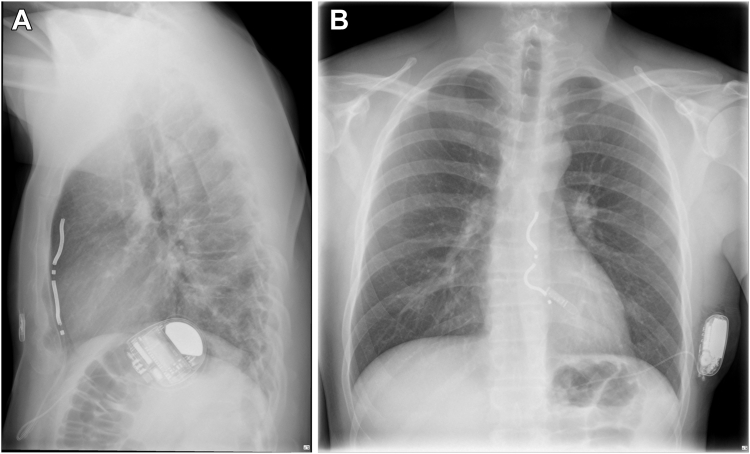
Radiograph after implant position lead and device of an Aurora extravascular implantable cardioverter-defibrillator in anteroposterior and lateral positions.
